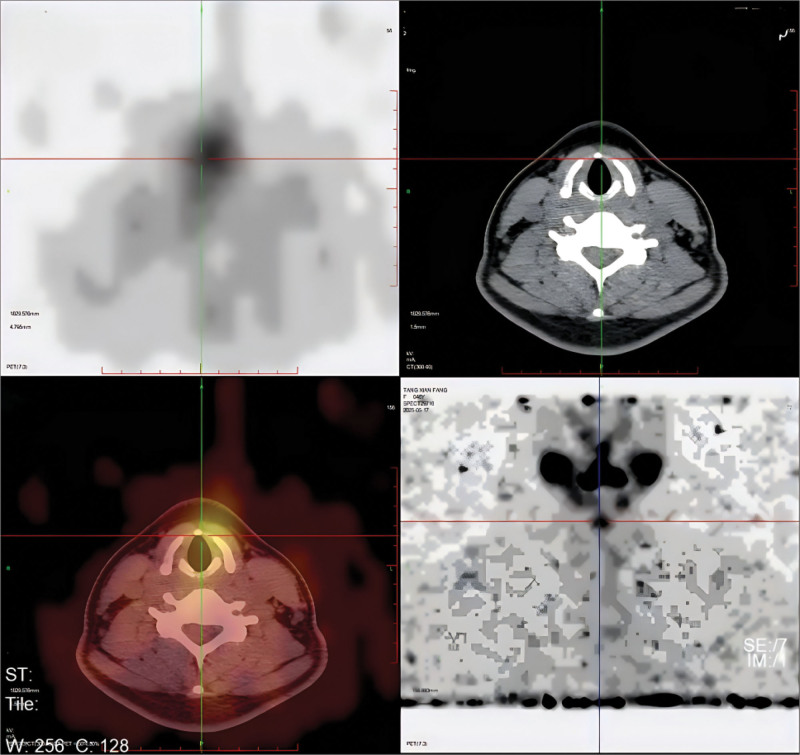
Fused ^131^I SPECT/CT at 48 hours demonstrates focal uptake anterior to the thyroid cartilage at the expected course of the thyroglossal duct (crosshair), with no corresponding soft-tissue lesion on CT, consistent with a thyroglossal duct remnant. SPECT/CT = single photon emission computed tomography, computed tomography.
